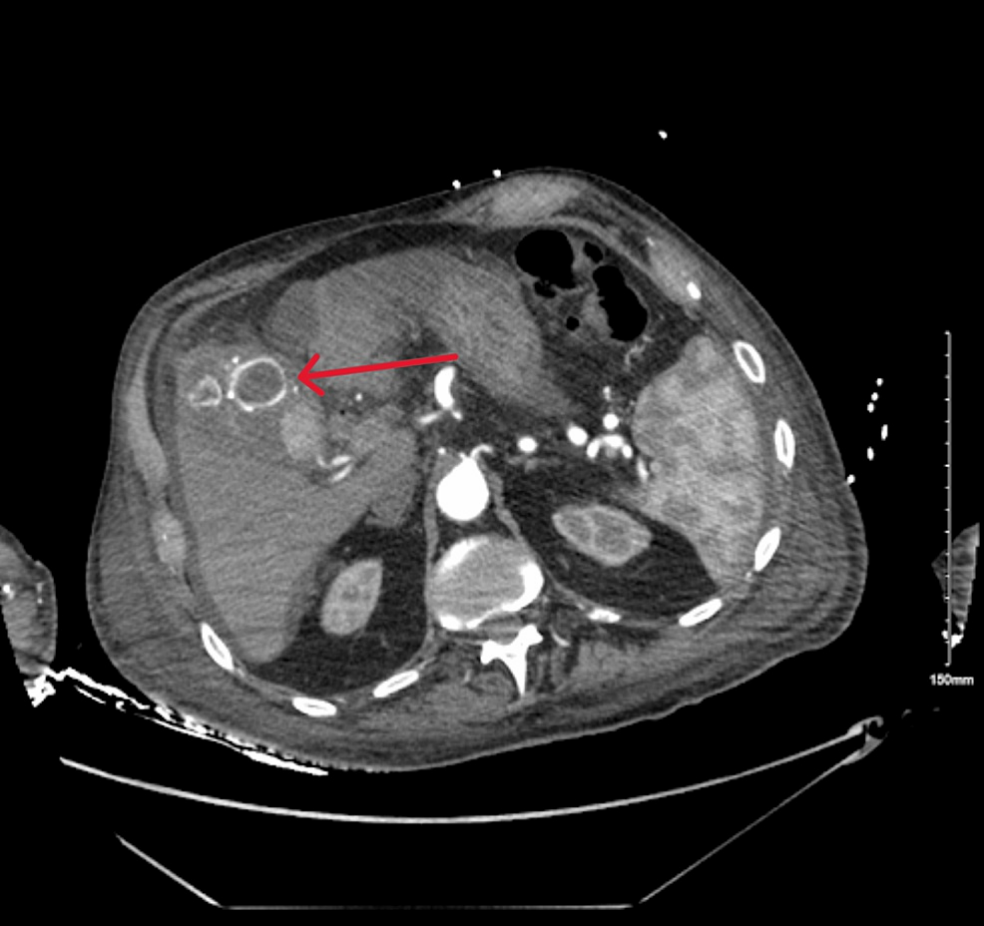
CT of the abdomen with contrast showing evidence of cholelithiasis and intrahepatic dilation.
